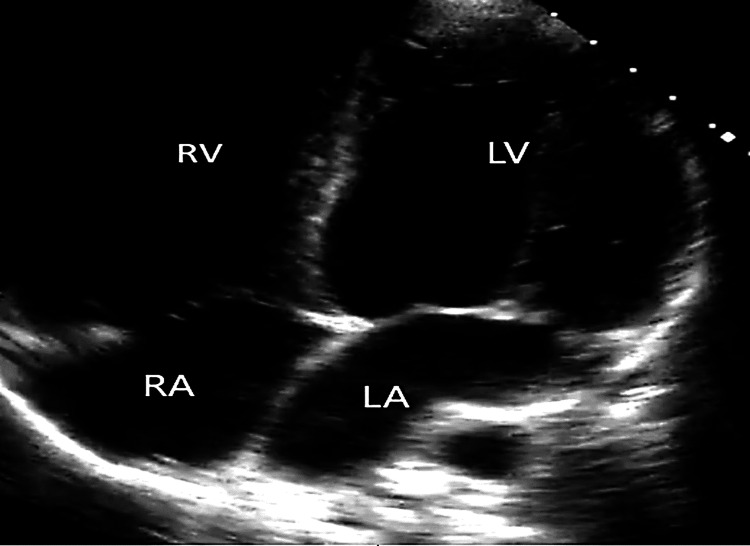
POCUS showing a dilated cardiomyopathy evident in the apical four-chamber view RV: right ventricle; LV: left ventricle; RA: right atrium; LA: left atrium
